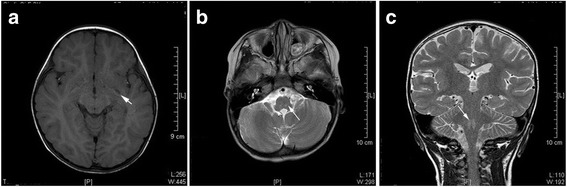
Abnormal MRI in intravalvarium. a Left basal ganglia (globus pallidus area) T1-WI low signal. b, c Bilateral bulbar (olive nuclear area) T2-MI high signal
